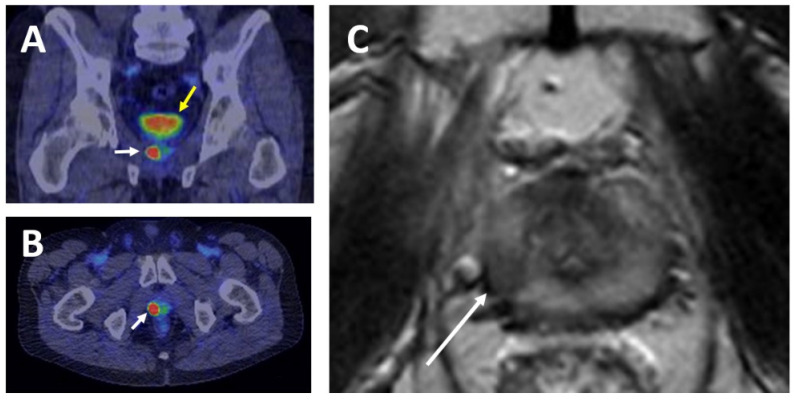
Example showing the potential of PSMA PET-CT imaging in detecting local recurrence of prostate cancer. Coronal (A) and axial (B) views of ^68^Ga-PSMA PET-CT demonstrate a recurrent right peripheral Gleason 5 + 5 carcinoma in a previously irradiated prostate (white arrow). The bladder (yellow arrow) is well visible. T2-weighted magnetic resonance imaging (C) indicates the presence of a PI-RADS (Prostate Imaging Reporting and Data System) grade 5 lesion or clinically significant cancer (white arrow) in the same area. Image: H. Minn, Turku PET Centre.
